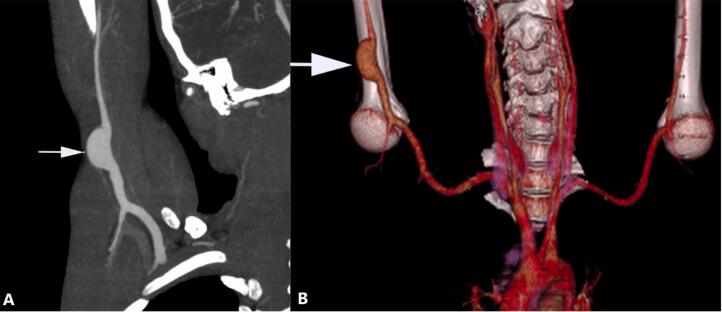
Multiplanar reconstruction (A) and volume rendering (B) images of CT angiography show a saccular aneurysm of the proximal brachial artery.
